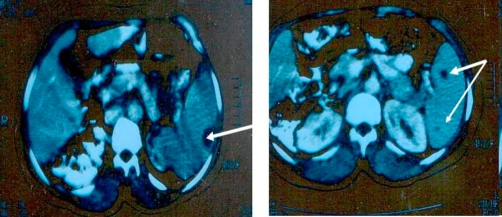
A pre-operative CT scan study showing well-defined focal lesions, cystic in nature and multiple in number located just subcapsular and deep in the splenic parenchyma.
